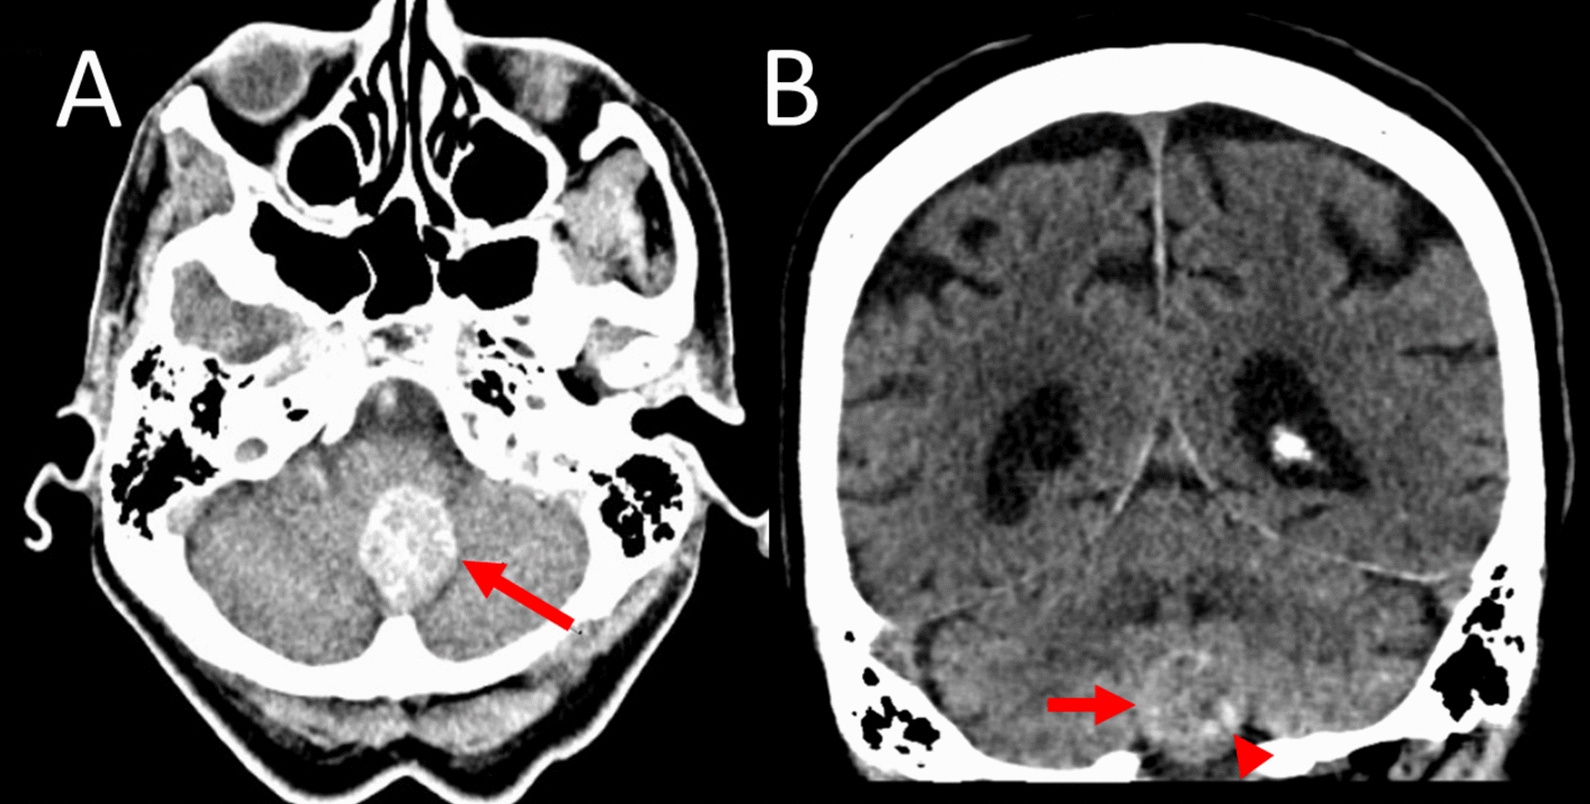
Infratentorial dorsal foramen magnum meningioma after 7 years and 3 months (cranial computed tomography). A Contrast medium-enhanced transverse-axial computed tomography section through the posterior fossa showing an avidly and homogeneously enhancing subvermal midline tumor (arrow) causing subpontine brain stem compression. B Transverse-axial computed tomography section without contrast enhancement showing the tumor (arrow) and inhomogeneous calcifications (arrowhead)
